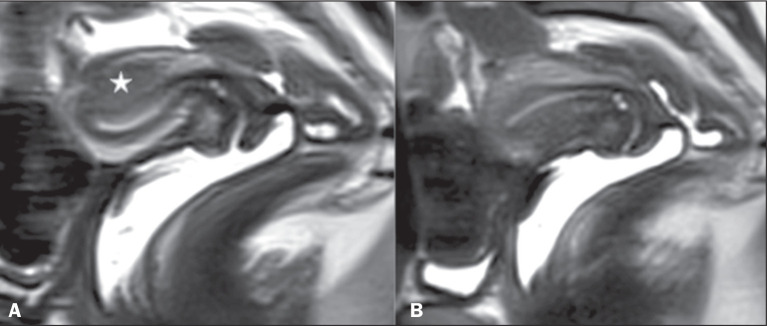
Sustained contraction: T2-weighted images in the sagittal plane. A:
